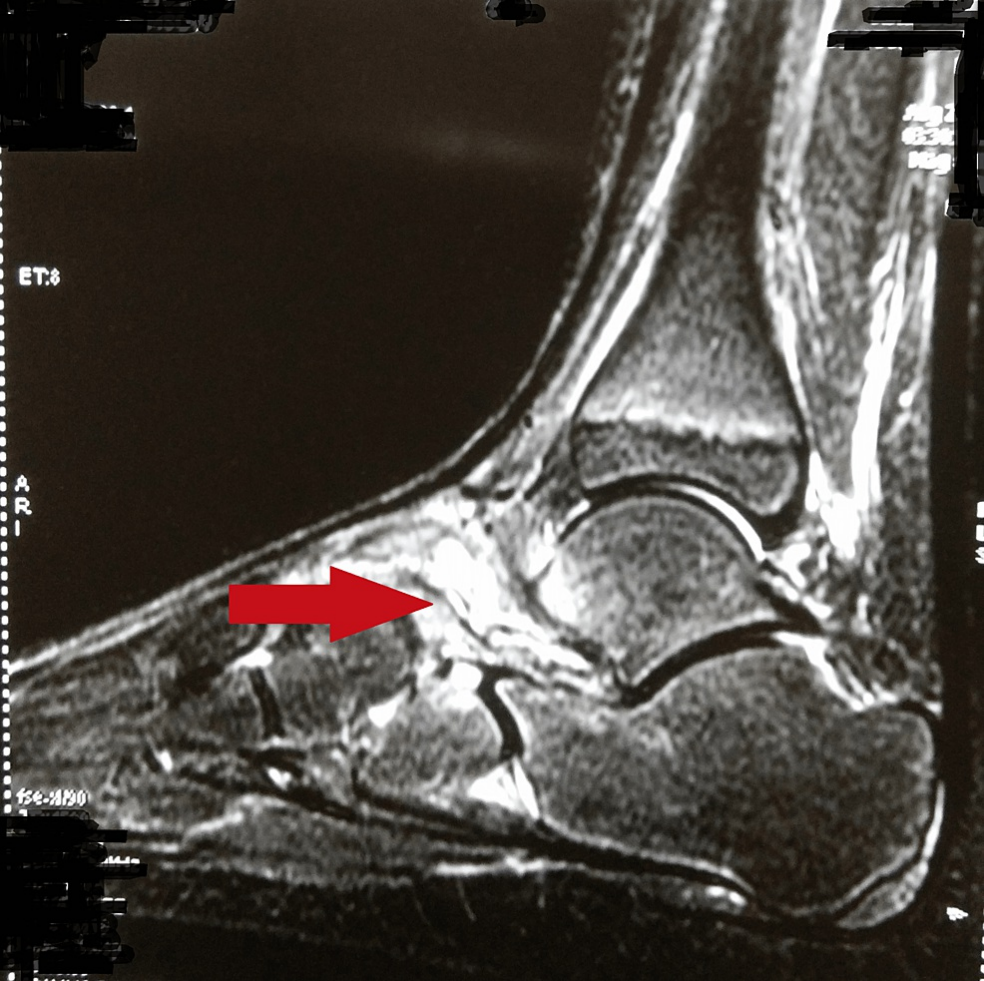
A magnetic resonance imaging of the right ankle suggestive of talus and navicular bones involvement with periarticular soft tissue collections
